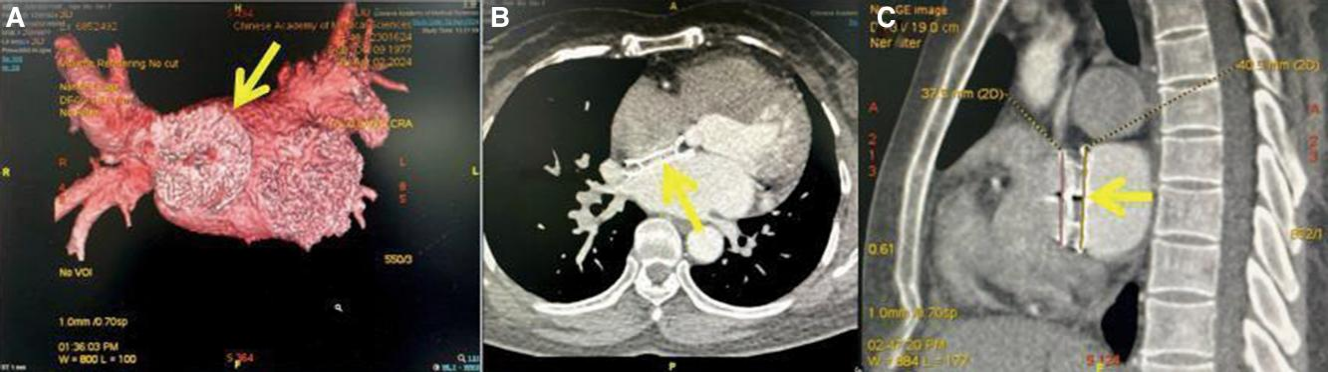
The arrows indicate a huge atrial septum occluder in the left atrial cardiac computed tomography: 3D reconstructions (*A*), transverse sections (*B*), and sagittal sections (*C*).
